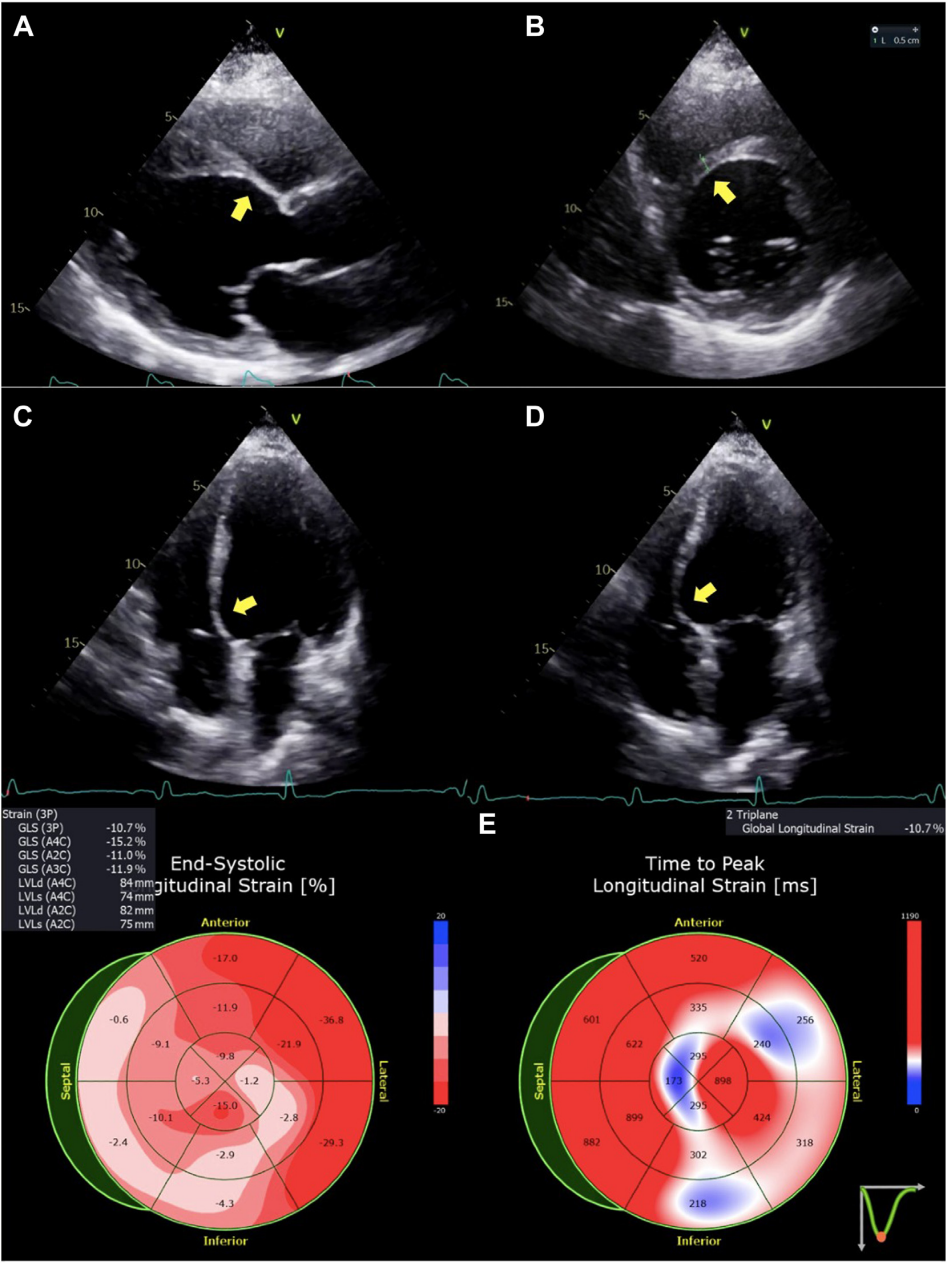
Two-dimensional transthoracic echocardiography, parasternal long-axis (A), parasternal short-axis (B) diastolic, apical four-chamber diastolic (C) and systolic (D) views, demonstrates a dilated, hypokinetic LV cavity with regional myocardial thinning and scarring of the basal segment of the IVS with aneurysmal motion *(arrows)*; the bull’s-eye display (E) of the end-systolic *(left)* and time to peak *(right)* LV global longitudinal strain patterns demonstrates significantly reduced values with greater regional impairment of basal segments of the IVS and inferior walls.
